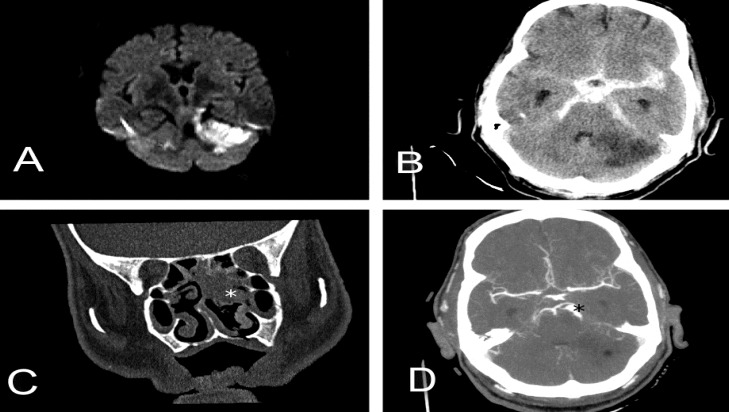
(A) Axial diffusion-weighted magnetic resonance imaging shows increased signal in left cerebellar hemisphere, representing acute ischemic infarction with restricted diffusion pattern, (B) axial computed tomography (CT) of the head shows extensive subarachnoid hemorrhage and cerebellar ischemic stroke, (C) CT coronal image shows an inflammatory process involving nasal cavity and ethmoid cells (white star), (D) Two consecutive fusiform aneurysms in superior cerebellar artery are evident in axial brain CT-angiography (black star).
